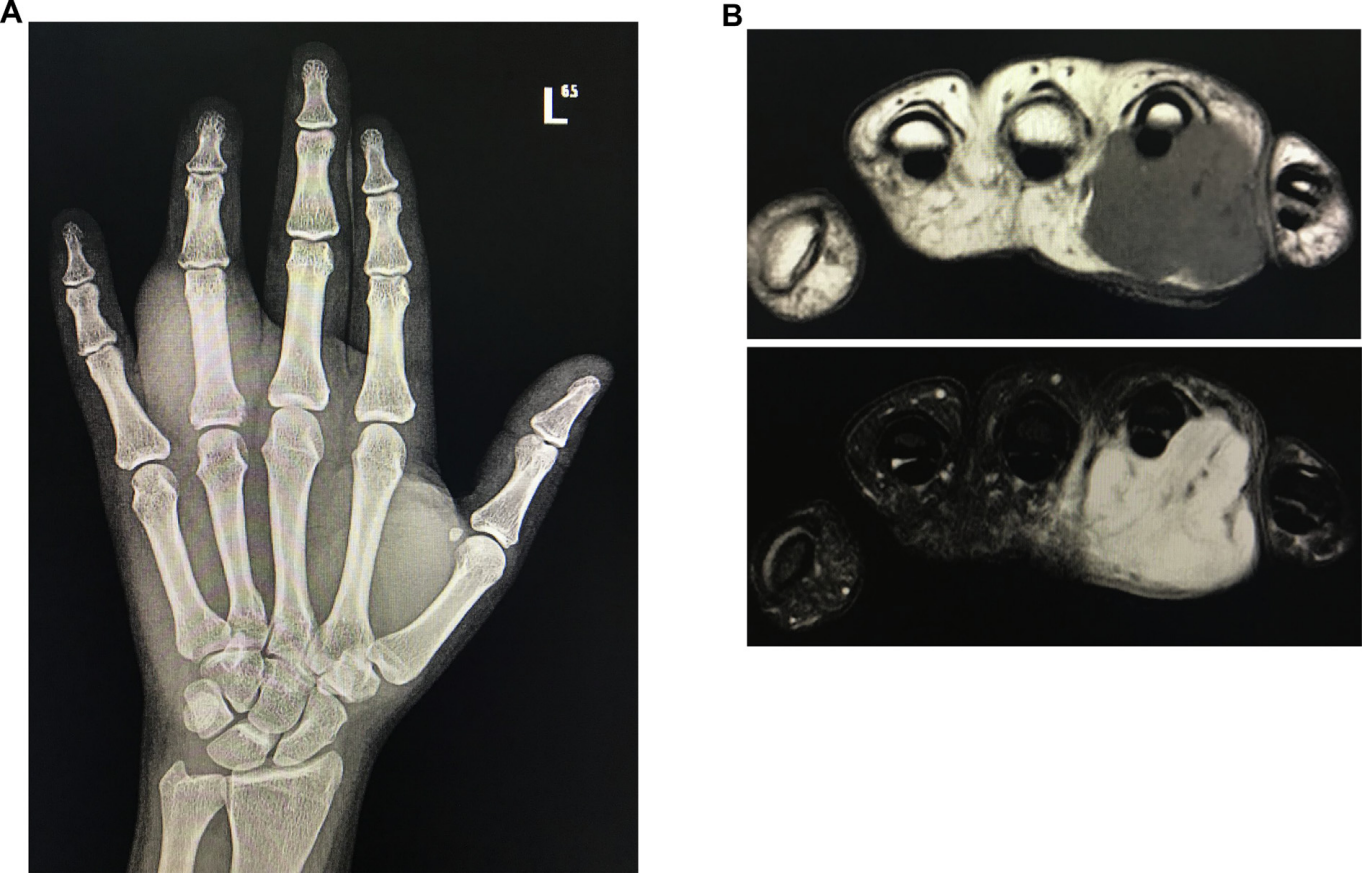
Pre-operative radiological investigation Fibro-osseous pseudotumor mass. A. It shows hand x-ray with extensive soft tissue swelling around the proximal phalanx of ring finger. B. It shows axial views of both T1-weighted and T2-weighted MRI imaging. Notice the extensive nature of the mass with involvement of neurovascular bundles.
